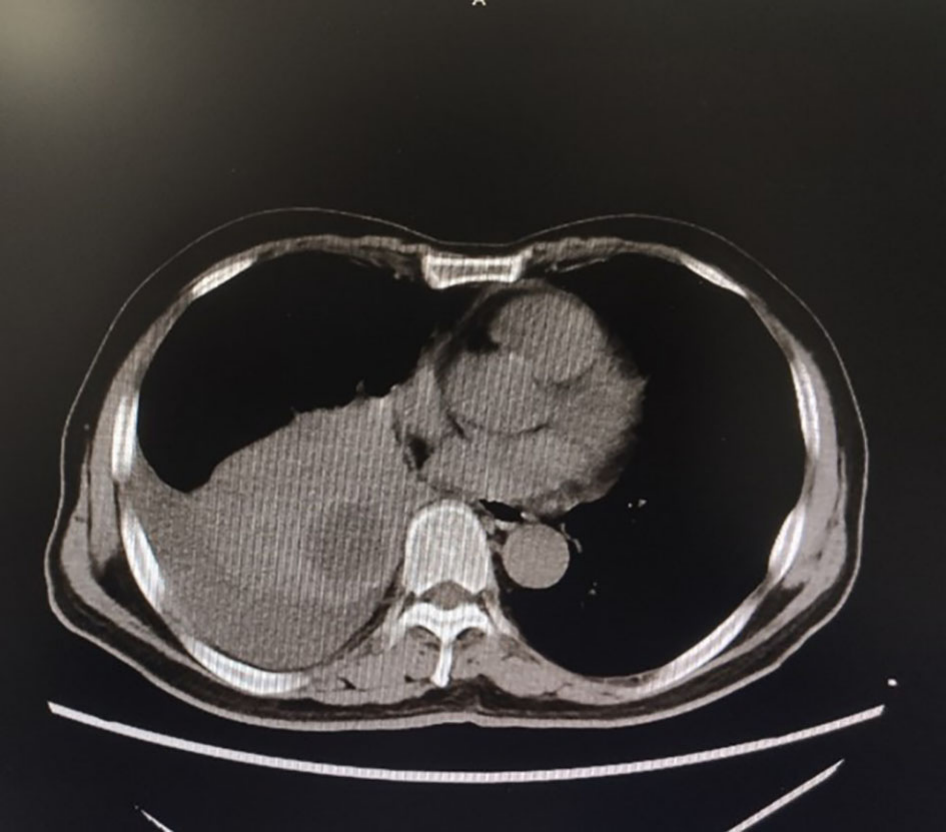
Chest CT for the first time in our hospital showed a space-occupying mass in the lower lobe of the right lung.
